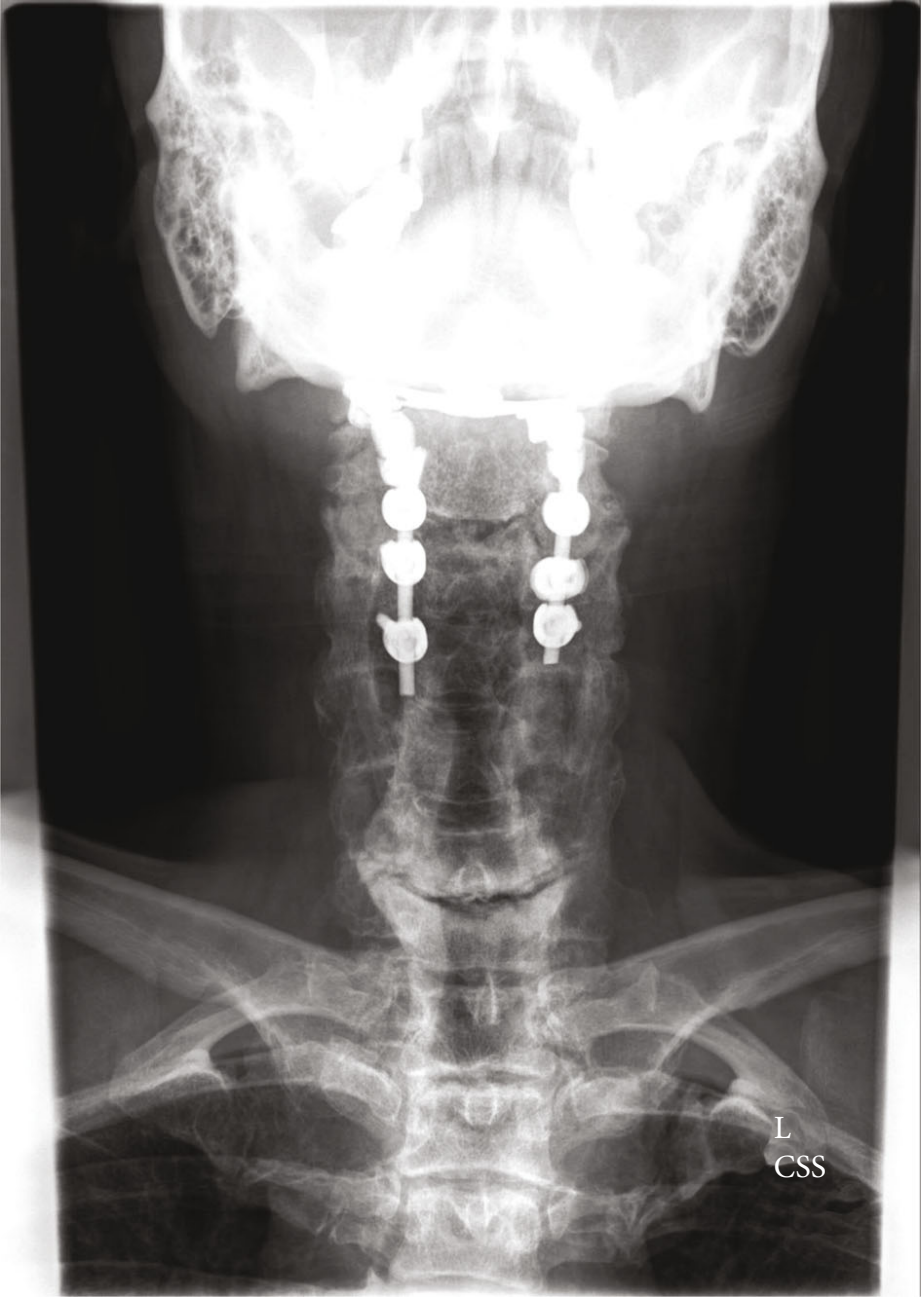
Postoperative anterior cervical spine X-ray demonstrates occipitocervical fusion with a C2-C4 lateral mass screw and posterior rod construct with the occipital plate.
